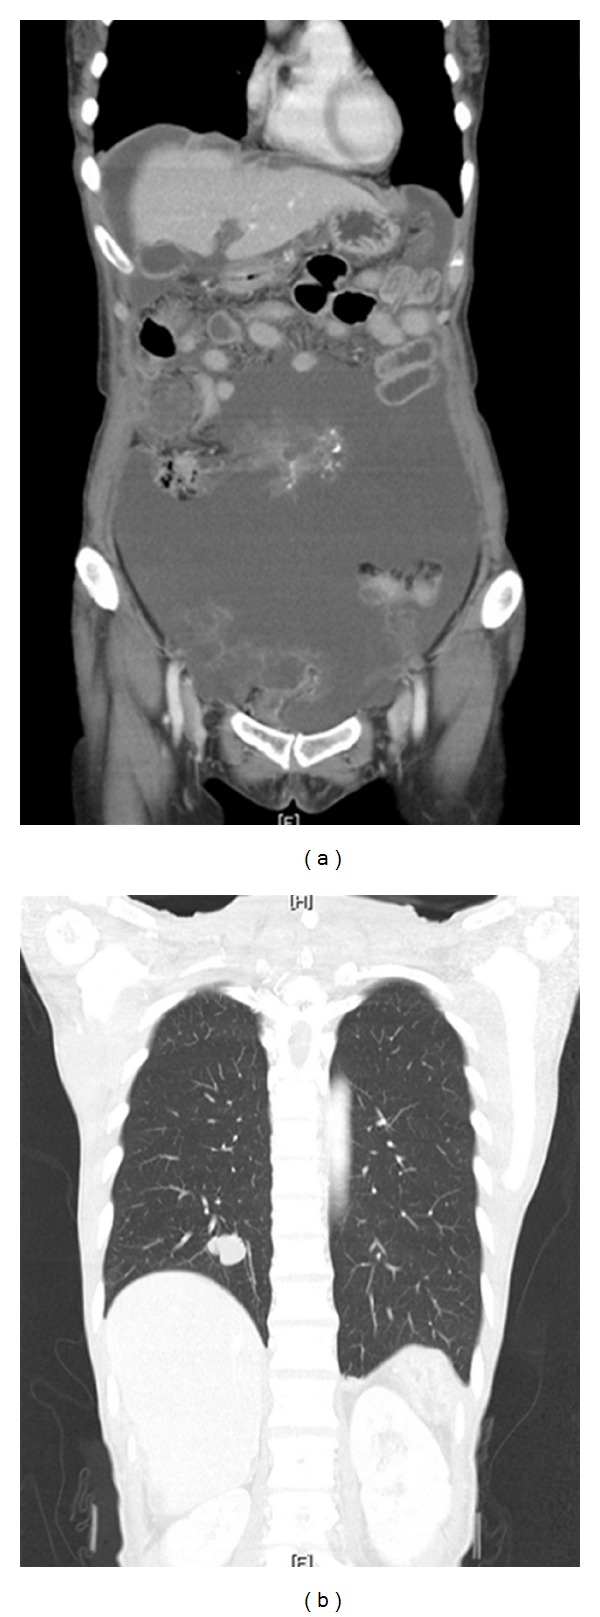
CT at the time of referral: massive tumor was diffusely spread in the peritoneal cavity. A solitary nodule in the right lower lung was also observed.
